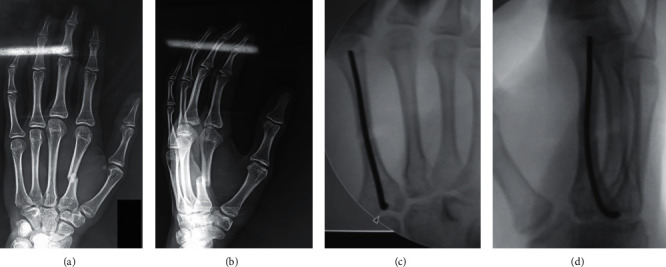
A 17-year-old male patient whose X-rays of anteroposterior and oblique views of the hand show a displaced diaphyseal fracture of the second metacarpal. Fluoroscopic photos show the final fixation of the displaced second metacarpal fracture with the three-point fixation of the intramedullary K-wire.
